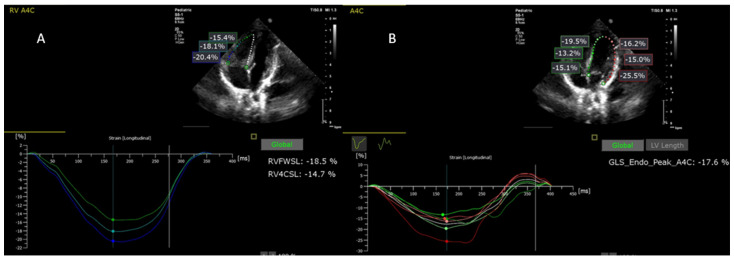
Biventricular speckle tracking analysis: (A) right ventricle speckle tracking analysis; RVFWSL—right ventricular free wall longitudinal peak systolic strain; RV4CSL—right ventricle four-chamber longitudinal strain; (B) left ventricle speckle tracking analysis; GLS_Endo_Peak_A4C (denoted by LV pGLS in our study)—peak global longitudinal strain of the left ventricle from apical four-chamber view.
